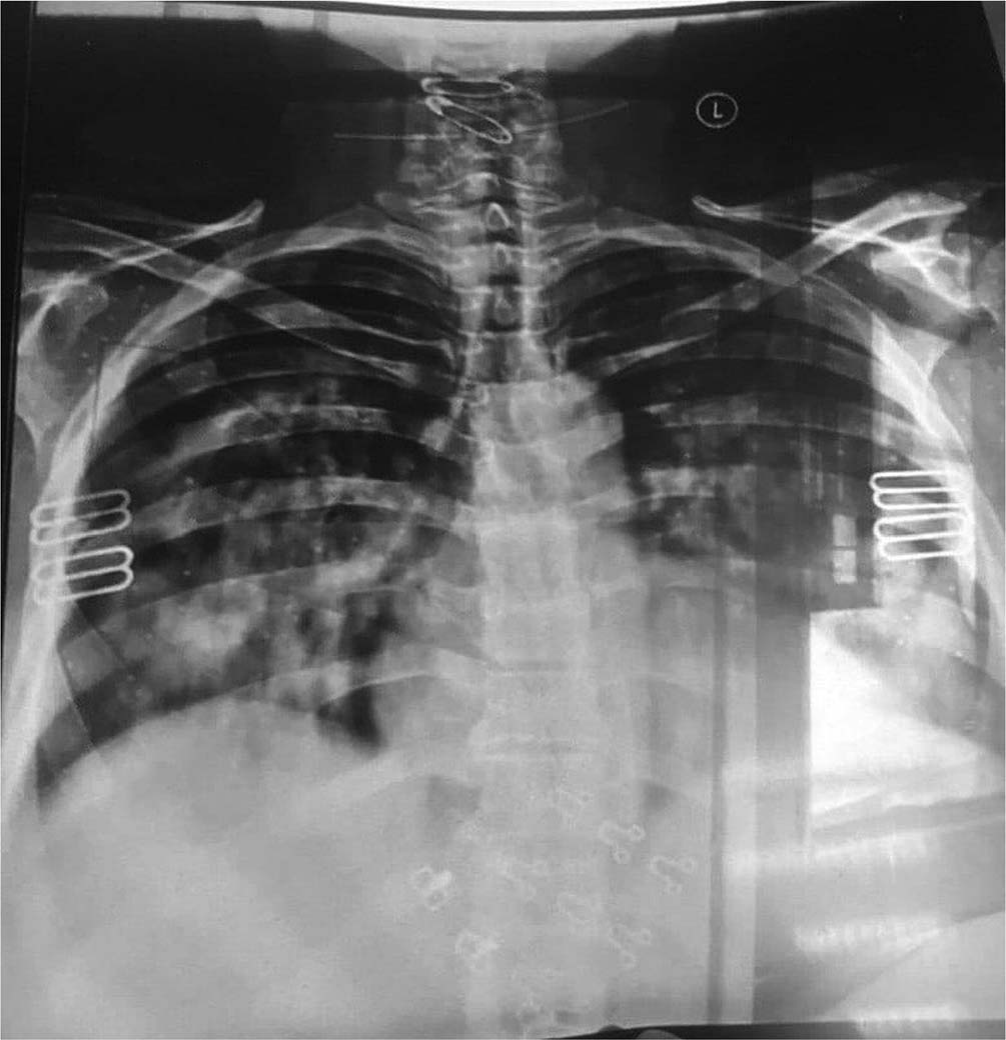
Chest radiograph shows: Bilateral diffuse infiltrates.
